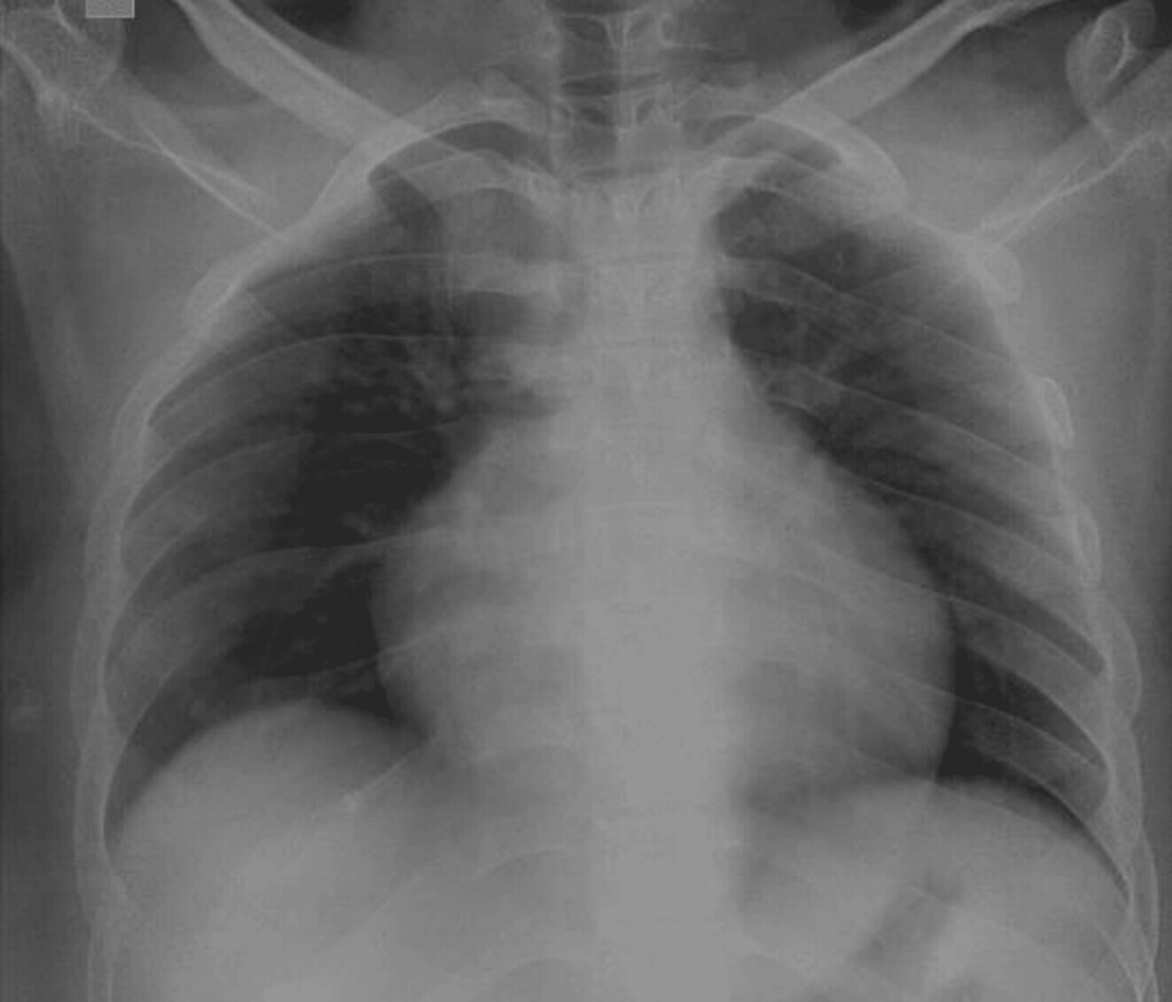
AP view of the chest X-ray shows cardiomegaly AP: Anteroposterior.
